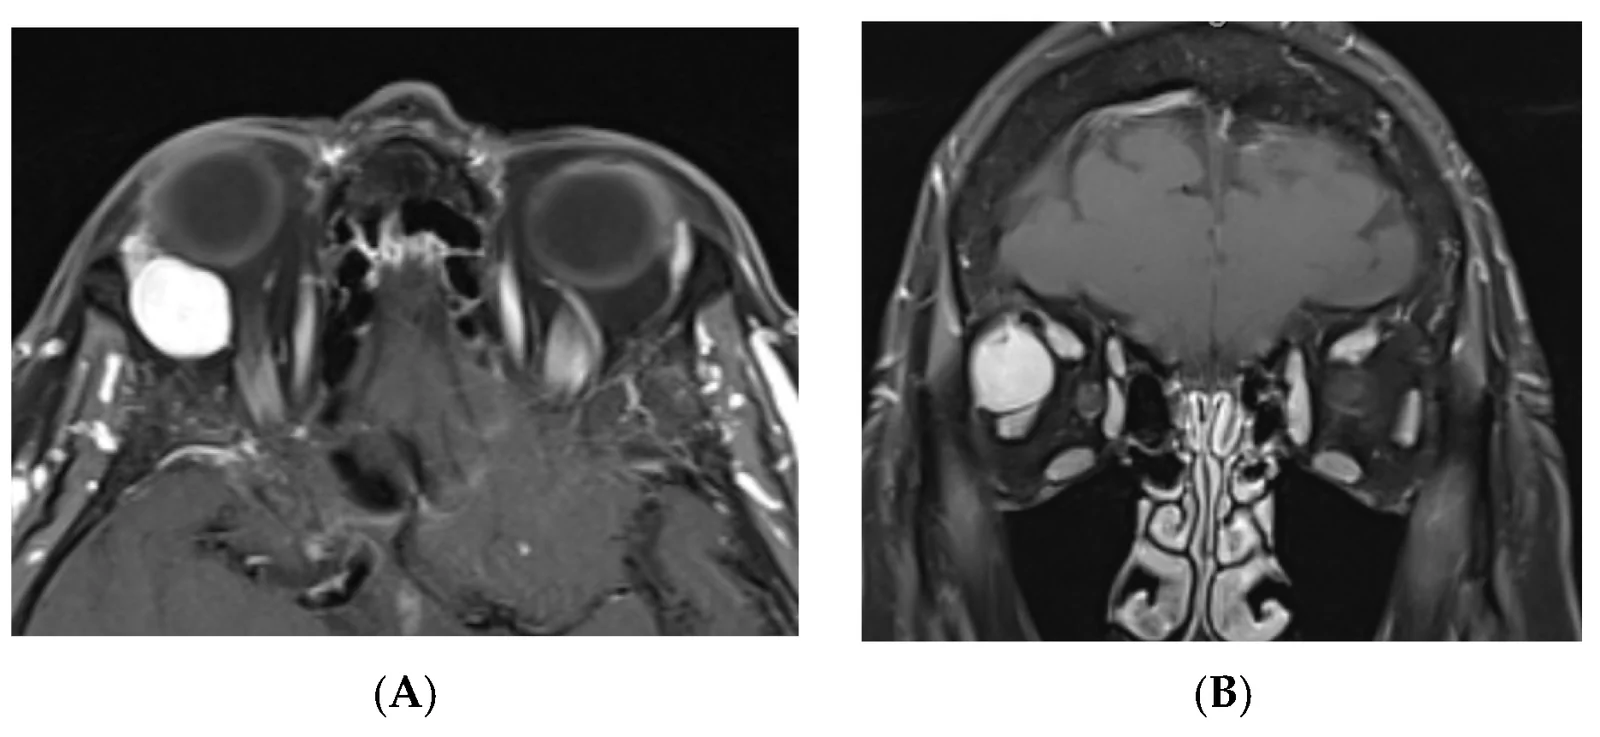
A 71-year-old woman presented with complaint of fullness in the upper eyelid without pain. (A,B) MRI images, axial (A) and coronal (B), T1 post-contrast images show a round, well-circumscribed lesion of the lacrimal gland. On gross total resection, the lesion turned out to be adenoid cystic carcinoma. The patient had post-op adjuvant radiation therapy.
